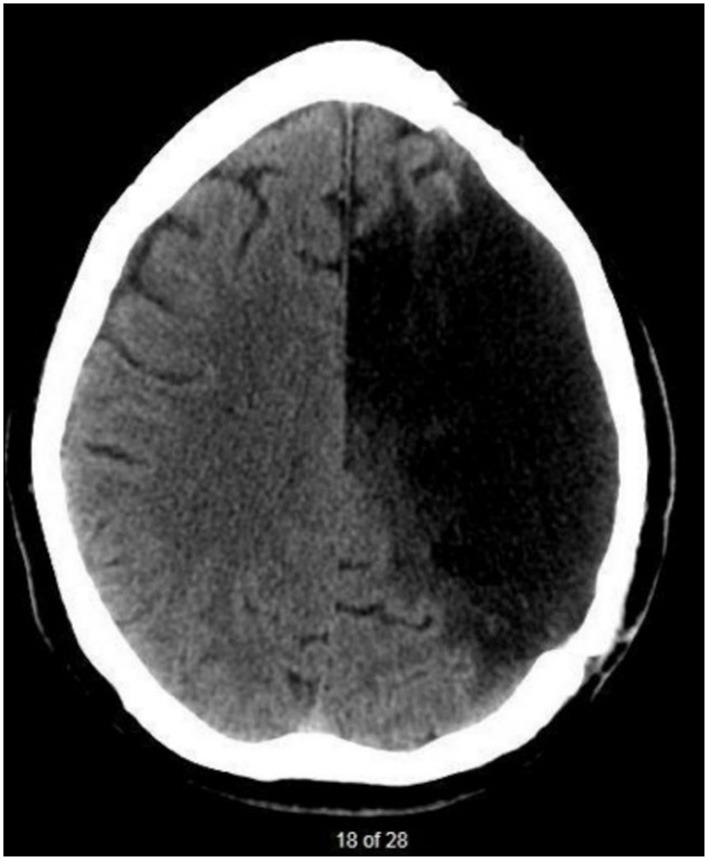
CT scan taken 3 years after JB's stroke. There is evidence of a large, chronic post-stroke lesion within the left-hemisphere. Regions affected are associated with branches of the left middle cerebral artery.
